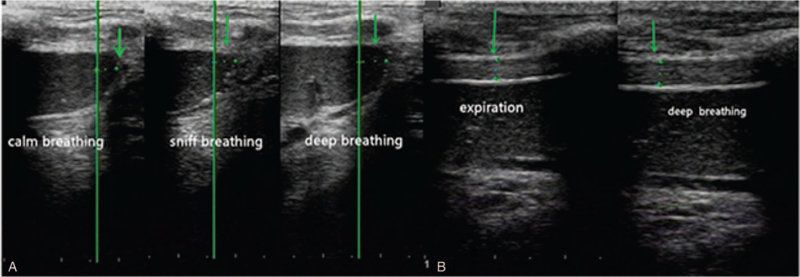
Ultrasonographic examination of the left diaphragm. A, The lower pole of the spleen moved 3.4 mm with calm breathing, 4.7 mm with deep breathing, and 3.7 mm with sniff breathing (green arrow). B, The left DT was observed by B-type ultrasound. The left DT was 2.9 mm at the end of expiration and 3.4 mm at the end of deep breathing (green arrow).
